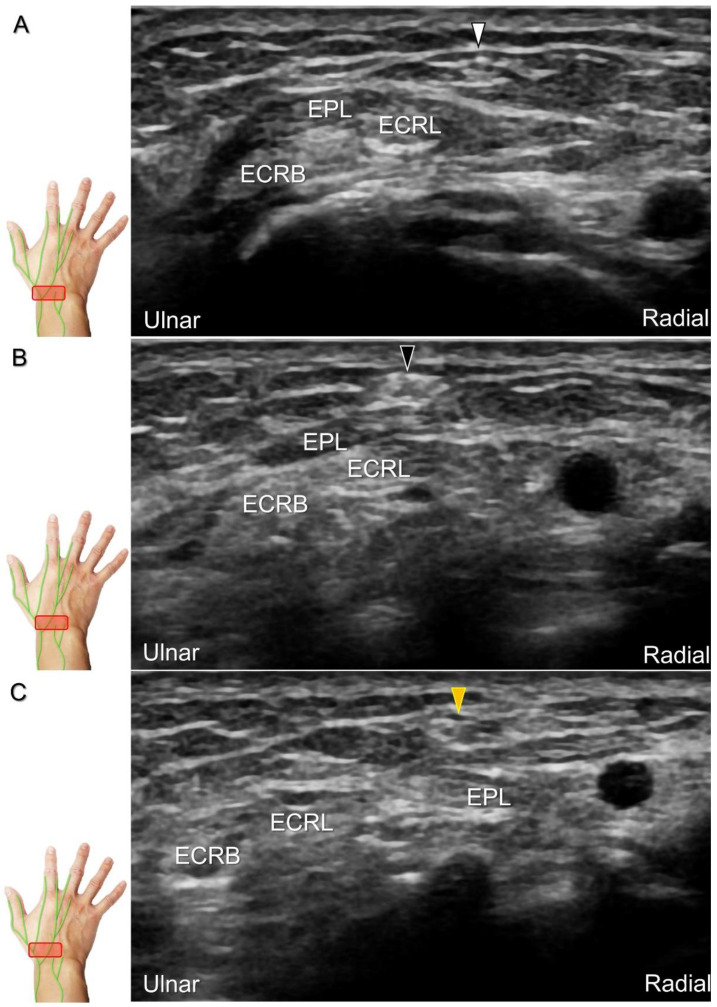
Sonographic imaging (short-axis view) of the dorsolateral branch of the superficial radial nerve at normal (A), swollen (B), and compressed (C) segments. White arrowhead: normal segment; black arrowhead: swollen segment; orange arrowhead: entrapped segment. ECRL: extensor carpi radialis longus tendon; ECRB: extensor carpi radialis brevis tendon; EPL: extensor pollicis longus tendon.
